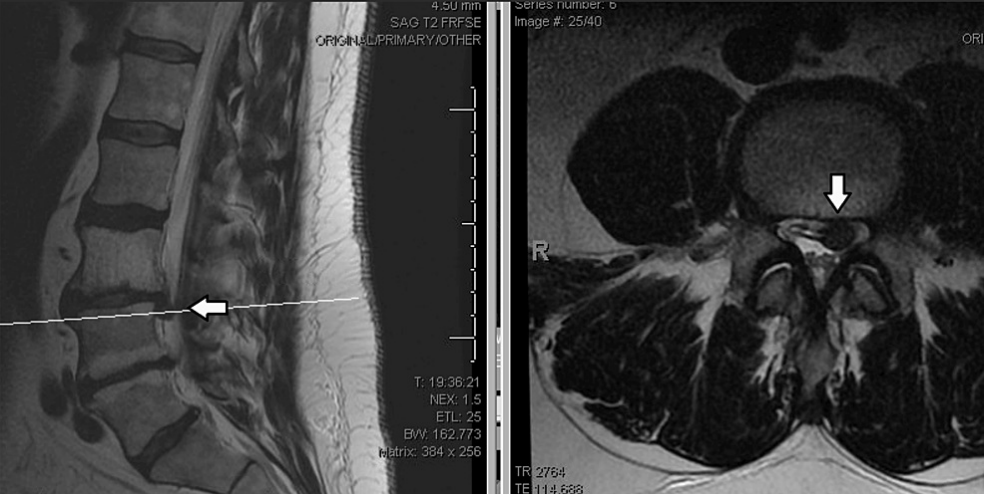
Pre-operative MRI Scan of Lumbar Spine, with Arrows Denoting L3/4 Disc Herniation Pre-operative MRI demonstrating multilevel spondylosis and a focal disc herniation at L3-4.
